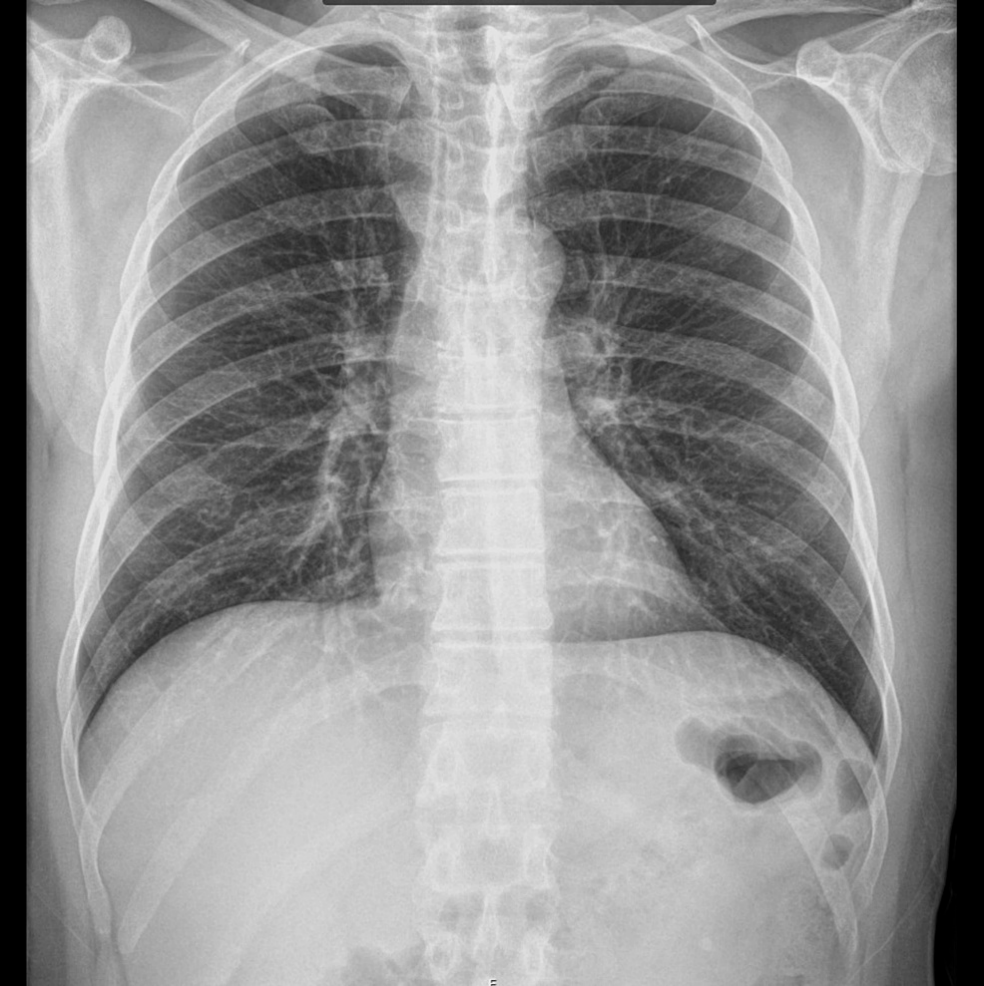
Chest X-ray on the first admission showing no significant alteration
